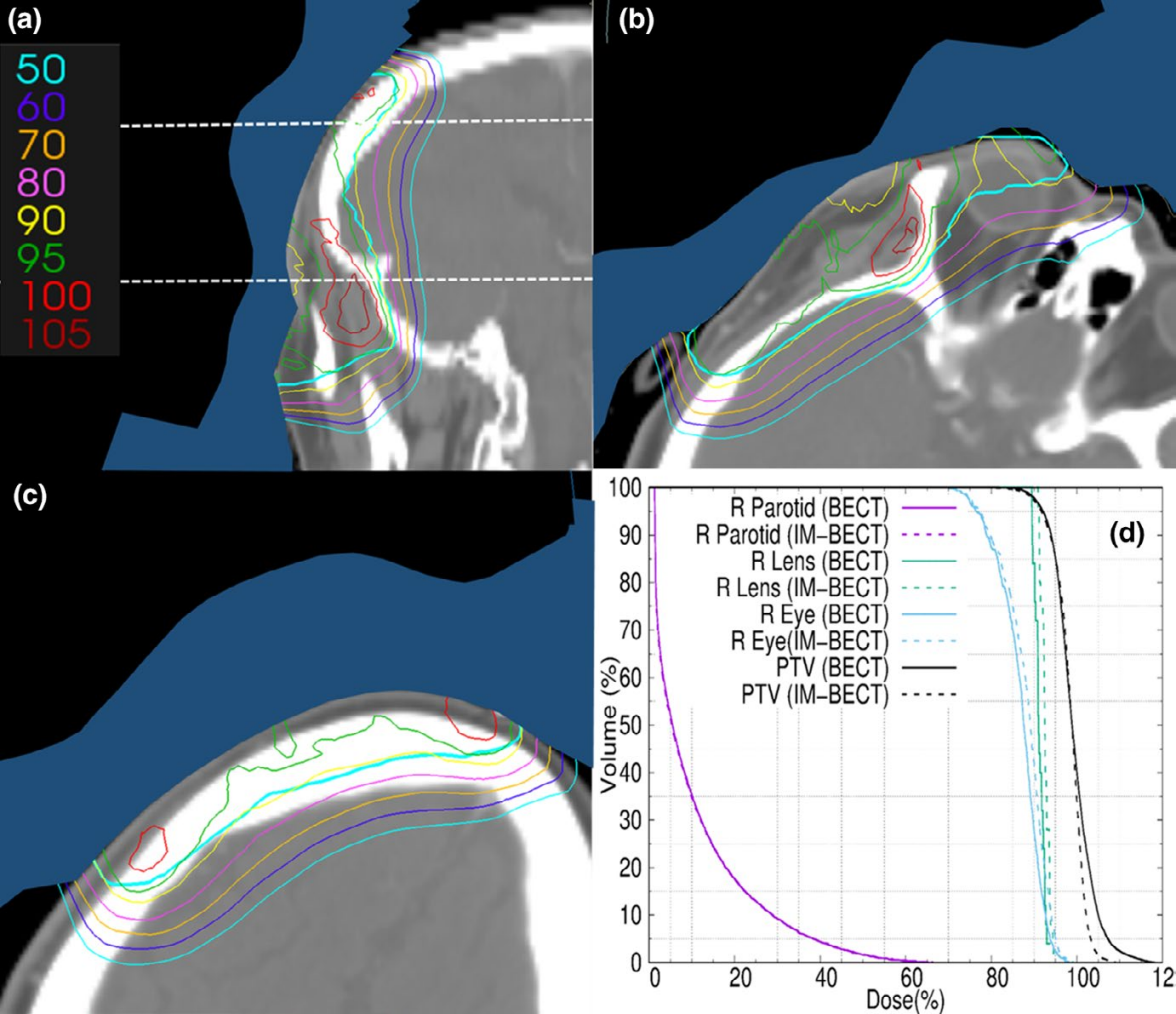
Temple patient CT planes showing the PTV (teal) and isodose lines (90% is yellow) for IM‐BECT treatment plan. The bolus is shaded blue. (a) Sagittal plane containing the central axis of the beam; dashed lines indicate positions of axial planes. Axial planes are (b) 3.0 cm inferior (R eye and brain) and (c) 3.0 cm superior (brain) to the central axis of the beam. (d) PTV, right eye, right lens, and right parotid DVHs for IM‐BECT and BECT treatment plans
